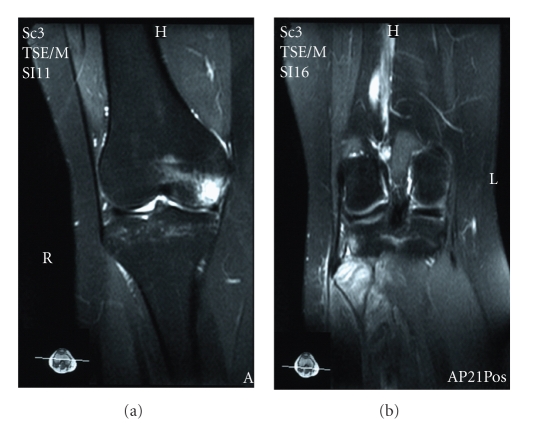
MRI of the right knee showing medial condyle bruise (a) as well as edema of the head of fibula due to fracture (b).
